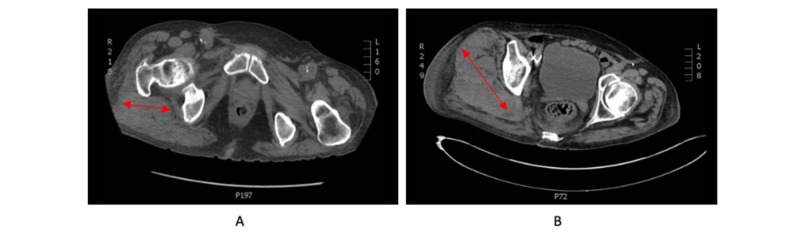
CT images of the right gluteal hematoma On initial admission, the right gluteal hematoma measured 3.8 cm x 3.1 cm (A) and on readmission, it measured 10 cm x 3.5 cm (B). CT: computed tomography
